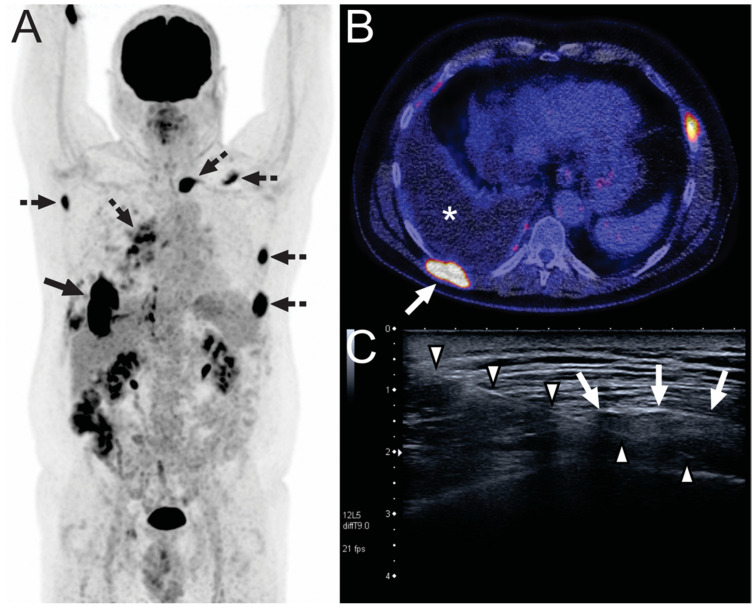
A 67-year-old man with stage IIIA right upper lobe lung adenocarcinoma was treated with chemotherapy and radiation therapy. Four months after completion of therapy, a CT scan (not shown) suggested tumor progression with a right-sided pleural fluid collection, for which a (talc) pleurodesis was performed. Subsequently, he was referred to our tertiary care center for immunotherapy with crizotinib. Another four months later, FDG-PET was performed for response evaluation. FDG-PET (A) showed multiple FDG-avid pleural and extrapleural lesions (dashed arrows), the largest at the right dorsal side of the chest wall (continuous arrow), suspicious for metastatic disease. The largest extrapleural lesion on the right dorsal side of the chest wall is also seen on fused FDG-PET/CT ((B), arrow), along with a pleural effusion (asterisk). Pleural fluid aspiration showed a lymphocytic exudate without any malignant cells. Subsequently, ultrasound-guided biopsy was ordered by the pulmonary oncologist for lung cancer mutation analysis. The ultrasound image (C) shows the biopsy needle (arrowheads) piercing the extrapleural lesion on the right dorsal side of the chest wall (arrows). Pathologic examination revealed a multinucleated giant cell reaction due to talc particles and no lung cancer.
